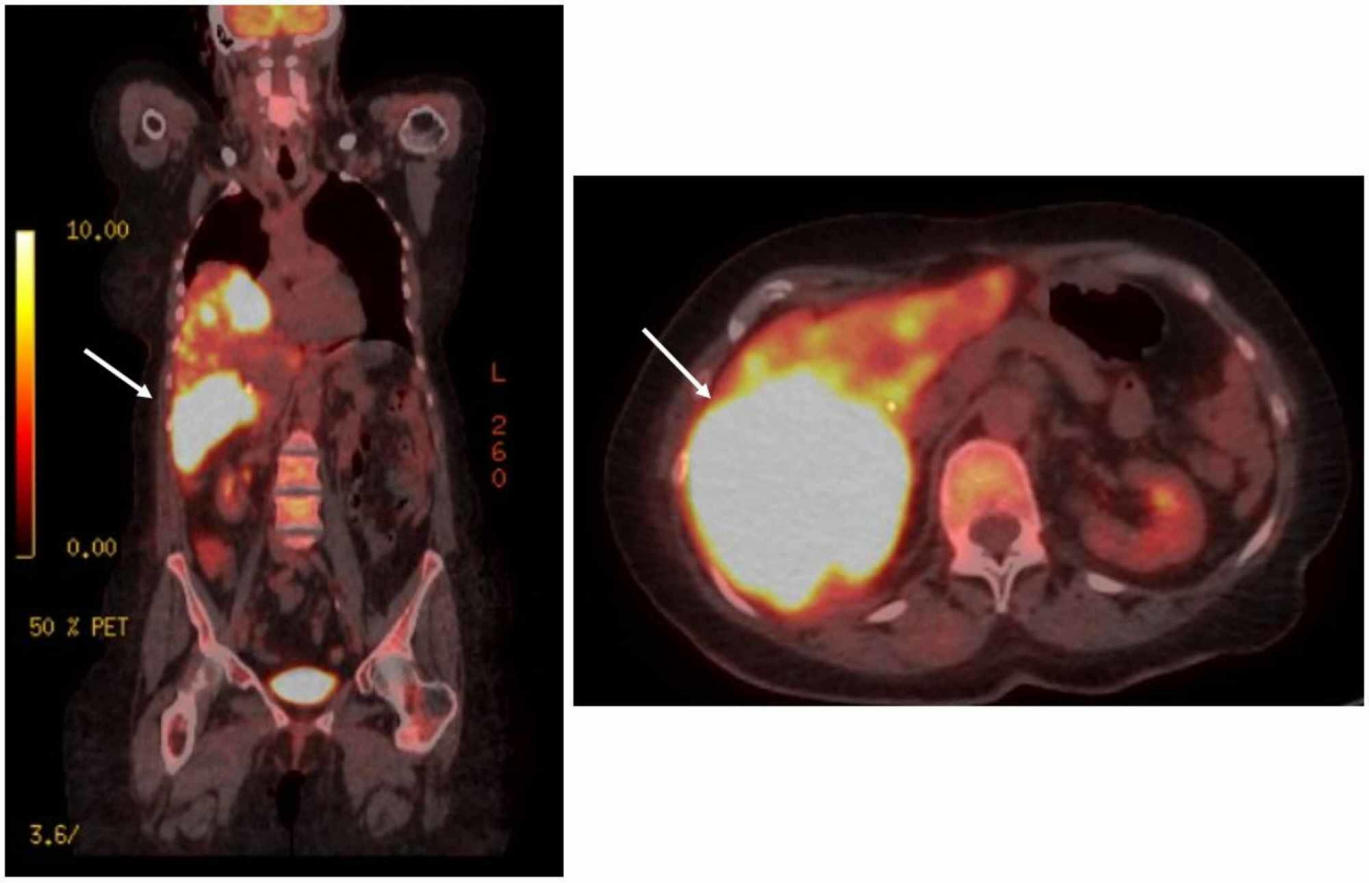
PET-CT: re-evaluation for metastatic disease Confirmation of metastatic disease, extensive liver involvement with multiple large hyperbolic hepatic metastases Right) Coronal view; Left) Axial view PET-CT: positron emission tomography-computed tomography
